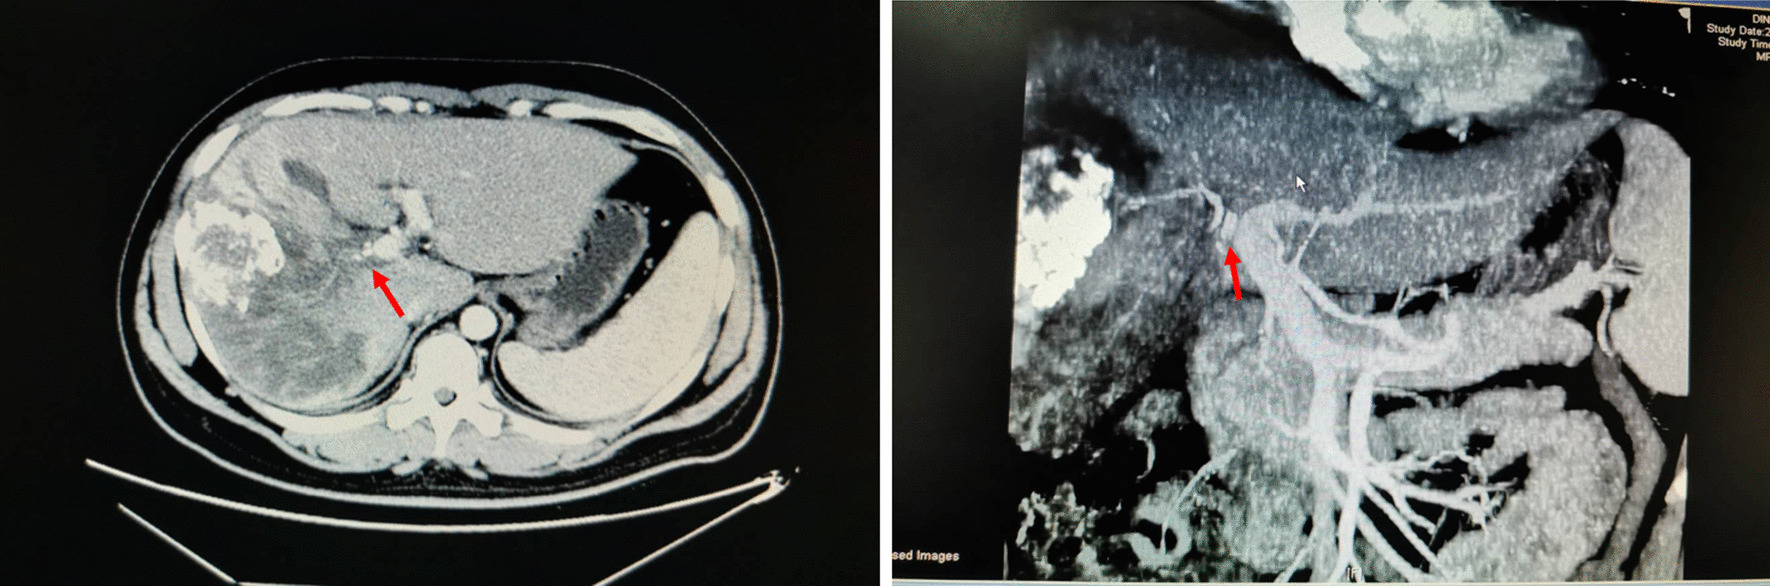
One week after the first postoperative operation, Computed Tomography scan of the liver showed that the right portal vein branch was occluded (red arrow). Moreover, part of the right liver presents a significantly low density, considering liver tissue necrosis
